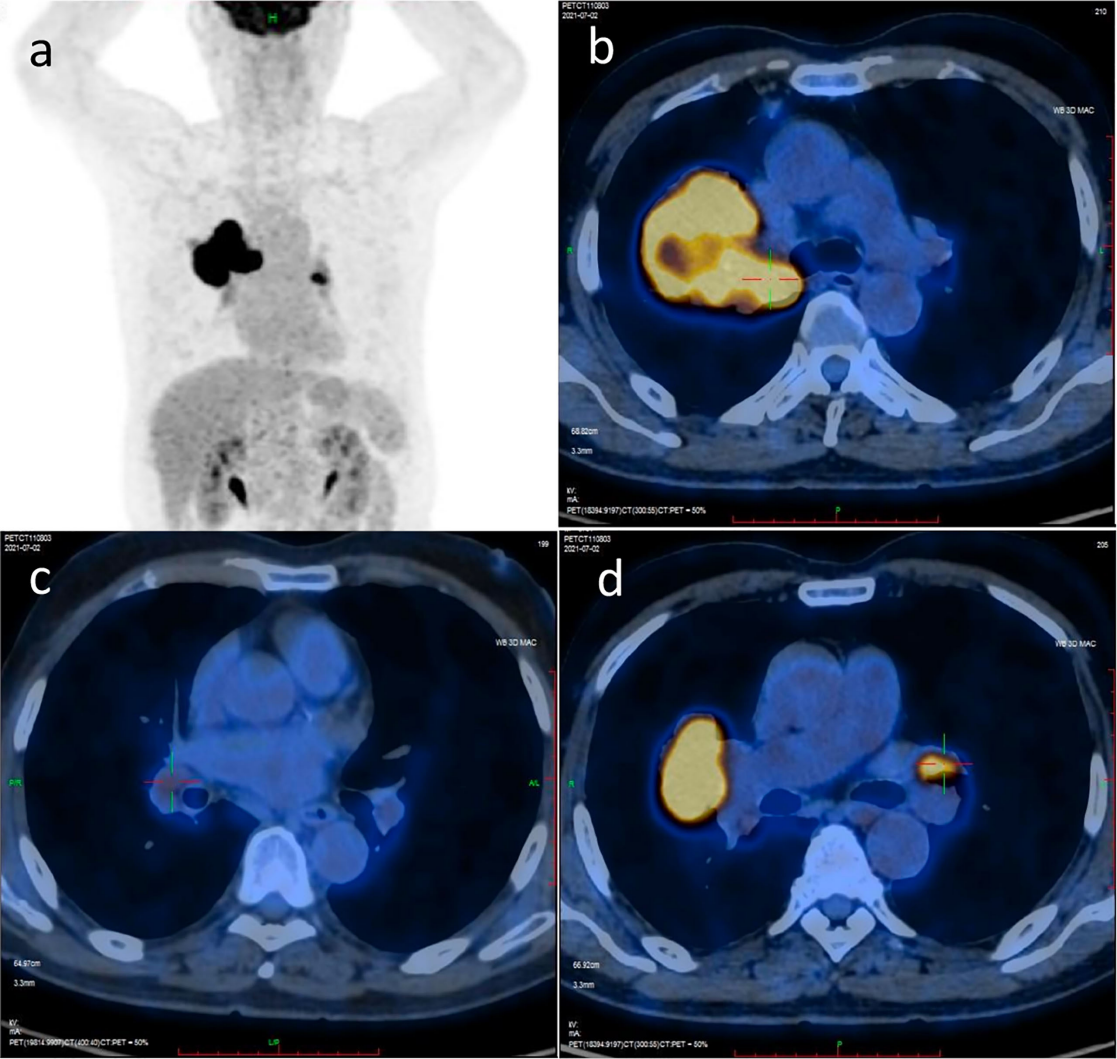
PET/CT scan image before treatment. (A) PET/CT scan image of chest and abdomen; (B) PET/CT scan image of irregular soft tissue mass in the right hilum; (C) PET/CT scan image of the enlarged lymph node in right hilar; (D) PET/CT scan image of the enlarged lymph node in left hilar.
